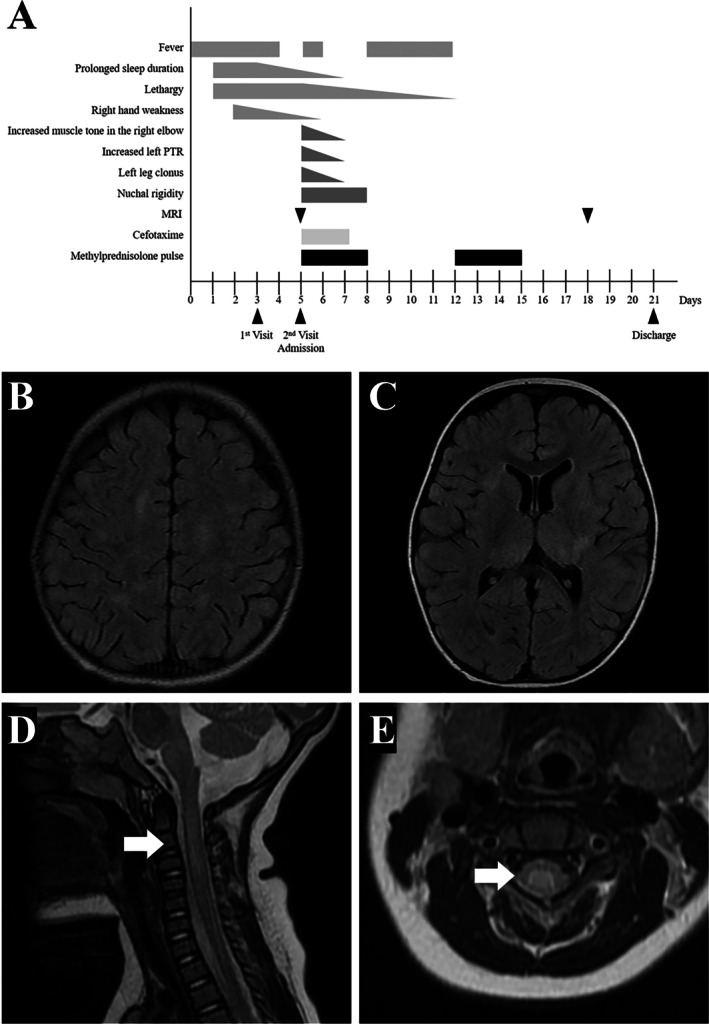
(A) Clinical course of the patient. Horizontal bars indicate the duration of symptoms and treatments. Fever, limb weakness, prolonged sleep duration, and lethargy were observed during the first week. At the second visit, he was still alert and able to walk but exhibited slightly increased muscle tone in the right elbow, left leg clonus, and hyperreflexia of the left patellar tendon. Methylprednisolone pulse therapy was administered twice (Days 5–7 and 12–14). MRI was performed on Days 5 and 18. The patient was discharged on Day 21. (B, C) Brain FLAIR images showing high‐intensity signals in the subcortical and deep white matter. (D, E) Cervical spinal cord MRI (T2‐weighted images) showing high signal intensity in the central gray matter at the C2–C6 levels (arrows).
